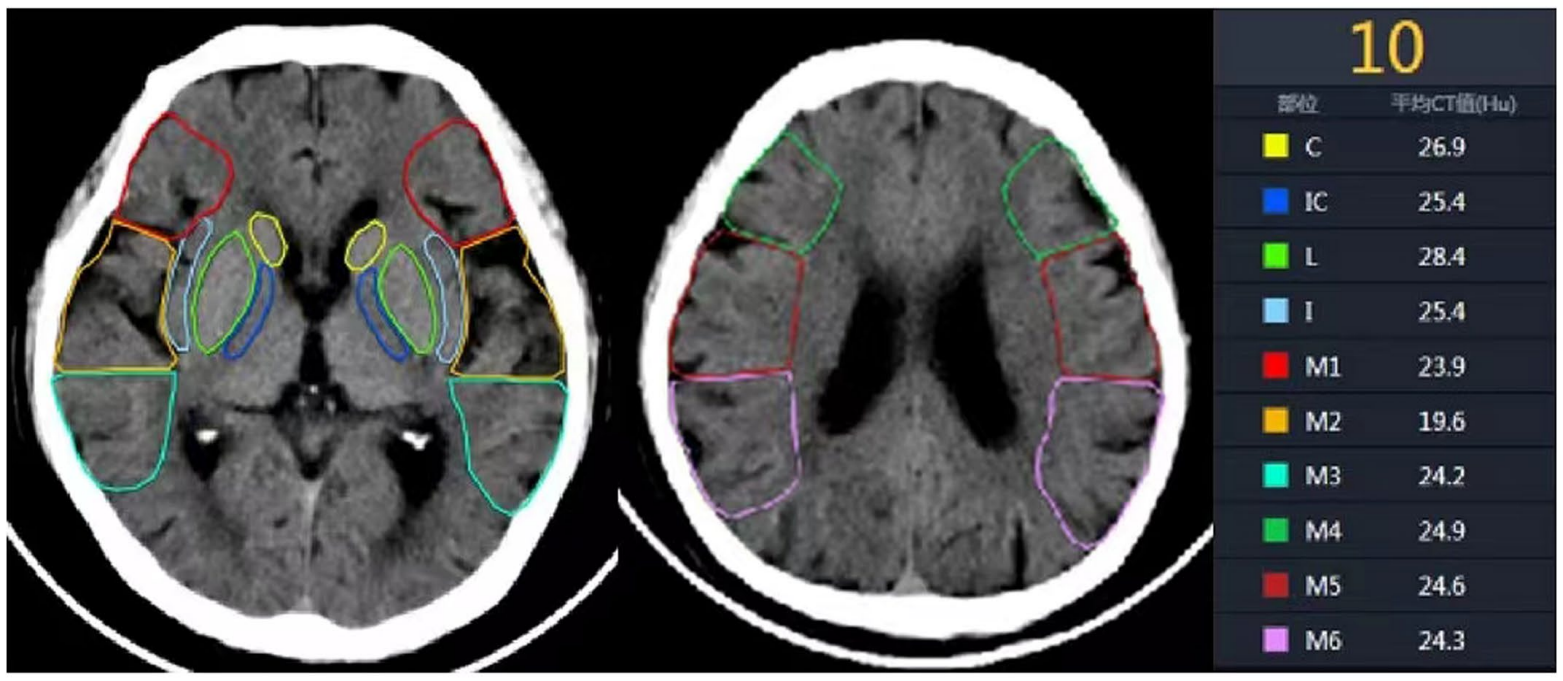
Ten anatomical regions of ASPECTS. C, Caudate nucleus; IC, Internal capsule; L, Lentiform nucleus; I, Insula; M1, anterior inferior frontal lobe; M2, temporal lobe; M3, inferior parietal and posterior temporal lobe; M4, anterior superior frontal lobe; M5, precentral and superior frontal lobe; M6, superior parietal lobe.
